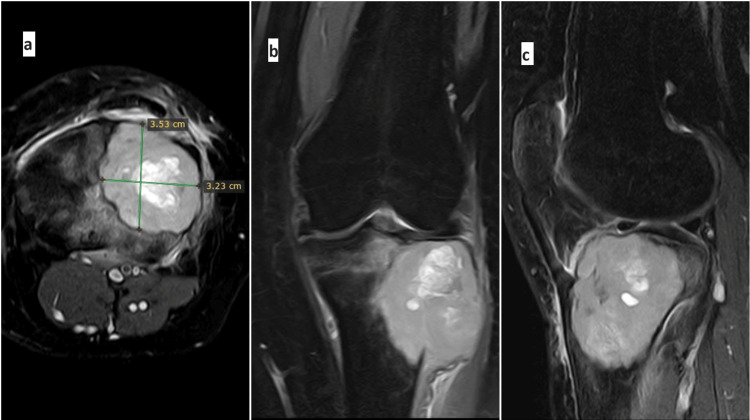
Preoperative evaluation and planning a: The size of the subchondral bone defect was measured in axial T2 images. (This can be done with CT axial images also.) A subchondral bone defect measuring 3.5 x 3.23 cm was found, which is the expected size of the inner column iliac crest graft to be taken. Exact measurements of the required graft will be taken intraoperatively after curettage of the lesion. b,c: Coronal and sagittal T2 MRI images showing the extent of the lesion CT: computed tomography; MRI: magnetic resonance imaging
